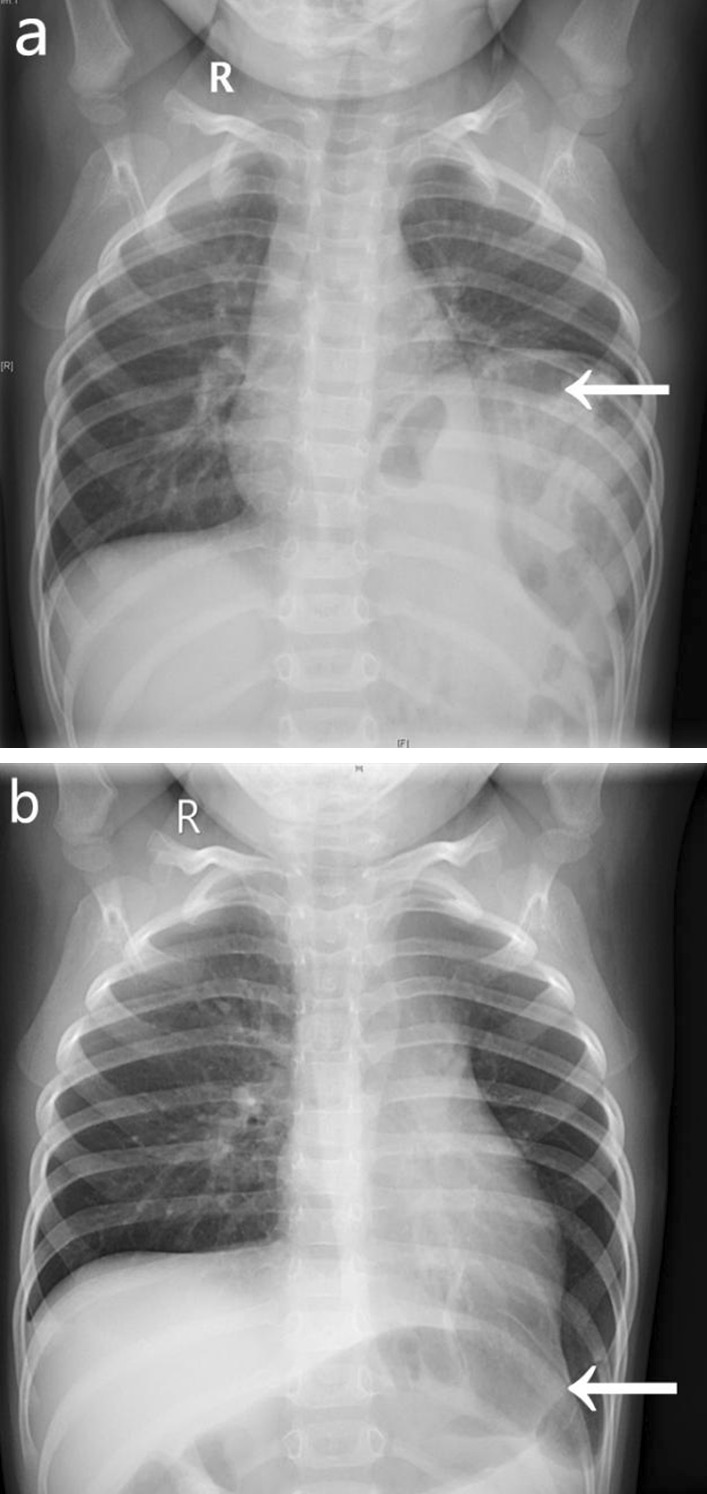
Preoperative chest radiograph of a child with CDE shows a raised left hemidiaphragm (a). A radiograph taken in the postoperative recovery period shows the left hemidiaphragm in the normal position (b)
